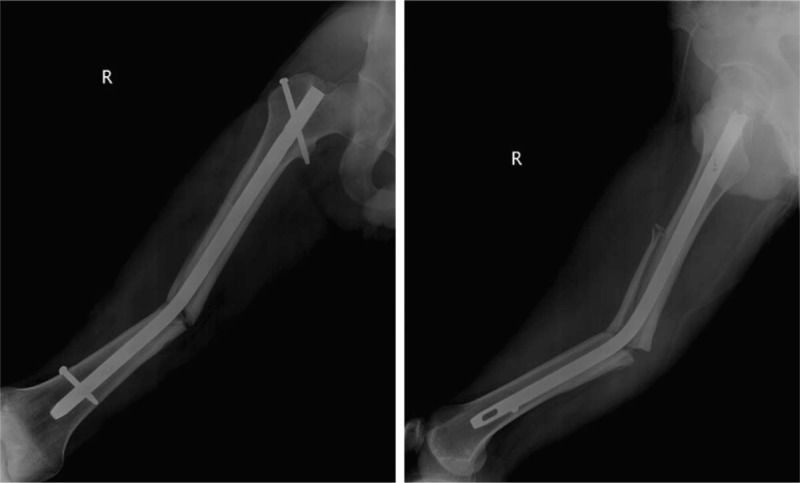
Radiographs showing bent IM nail with an apex-posterior angulation of 34.8° in the sagittal plane.
